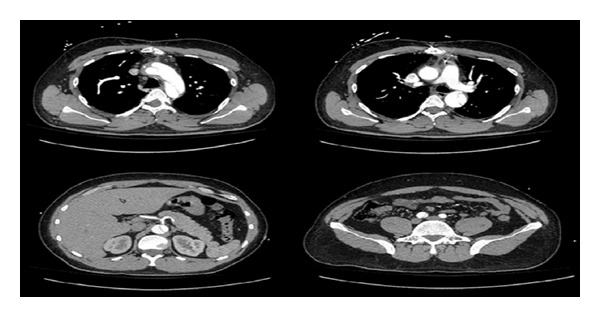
CT scan of second aortic aneurysm and dissection.
